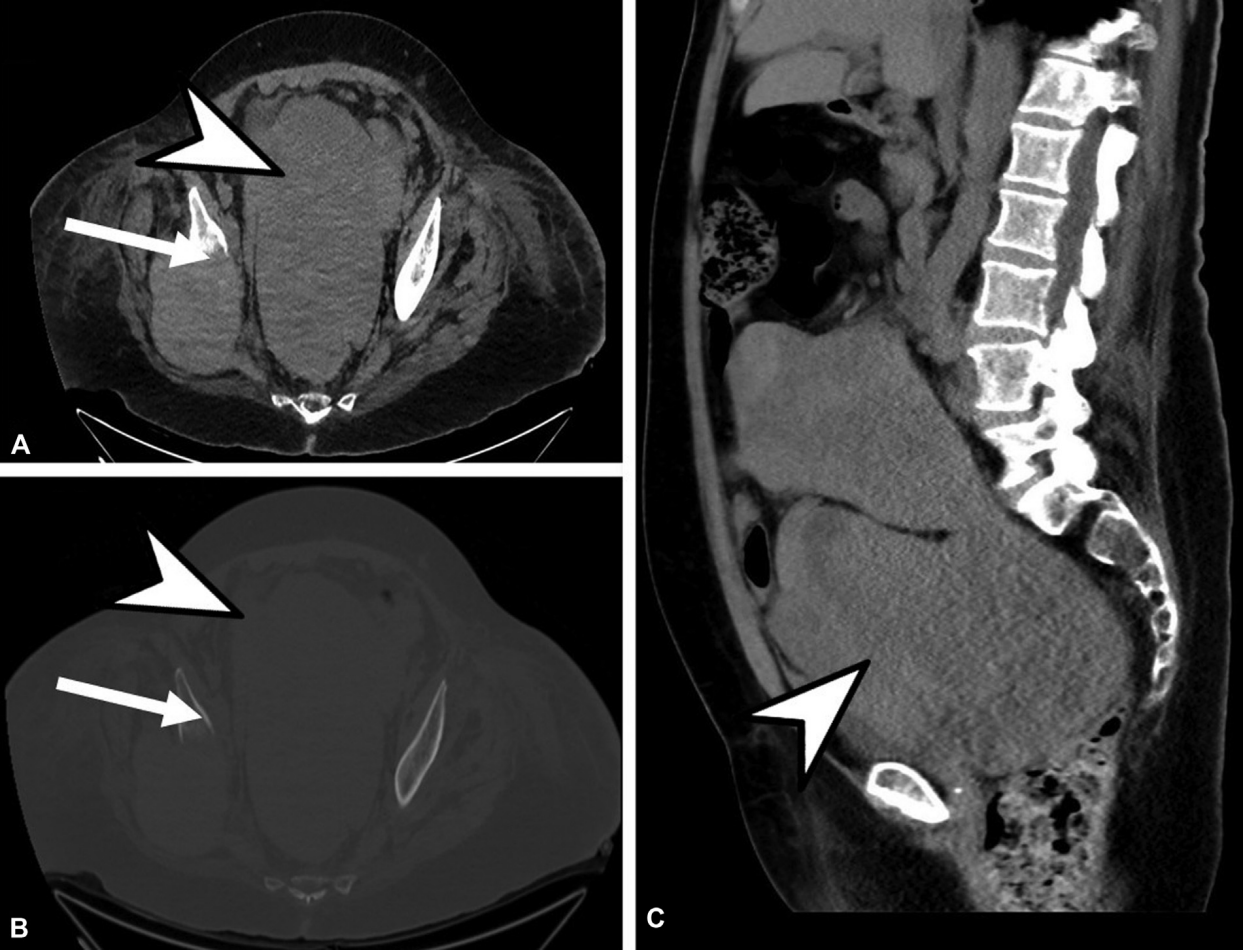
Abdominopelvic CT scan without contrast. (
